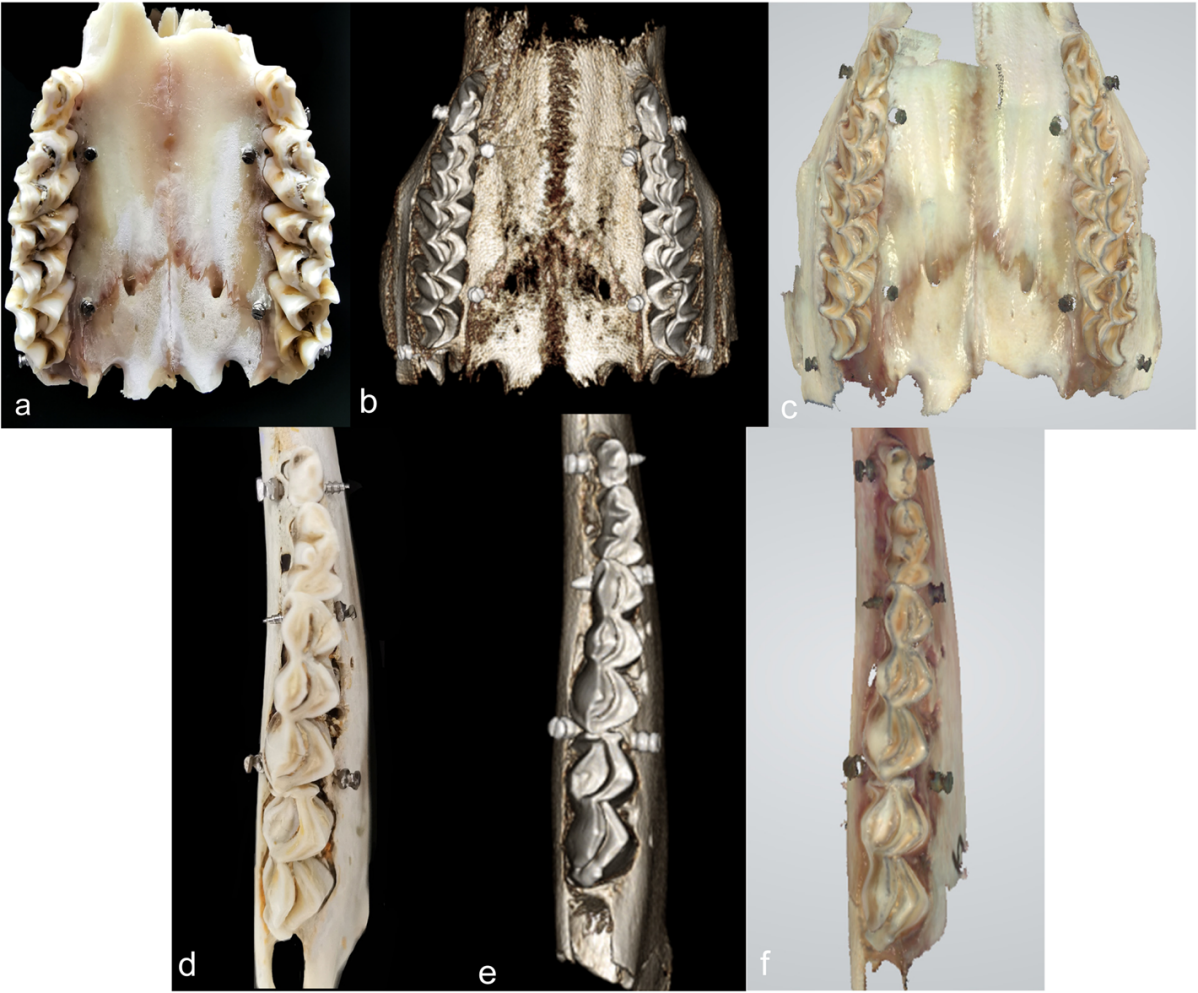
a and d Two representative images among 12 samples. b and e 3D models originating from CBCT of the two actual samples on the left side. c and f 3D models of the same samples on the left scanned from the IOS
